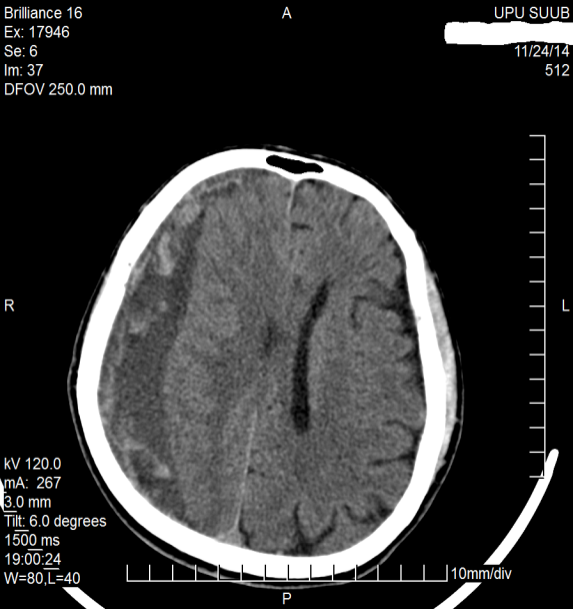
Postoperative CT aspect in patient B.I. of 71 years old; chronic subdural haematoma with a light recent bleeding of right brain hemisphere
